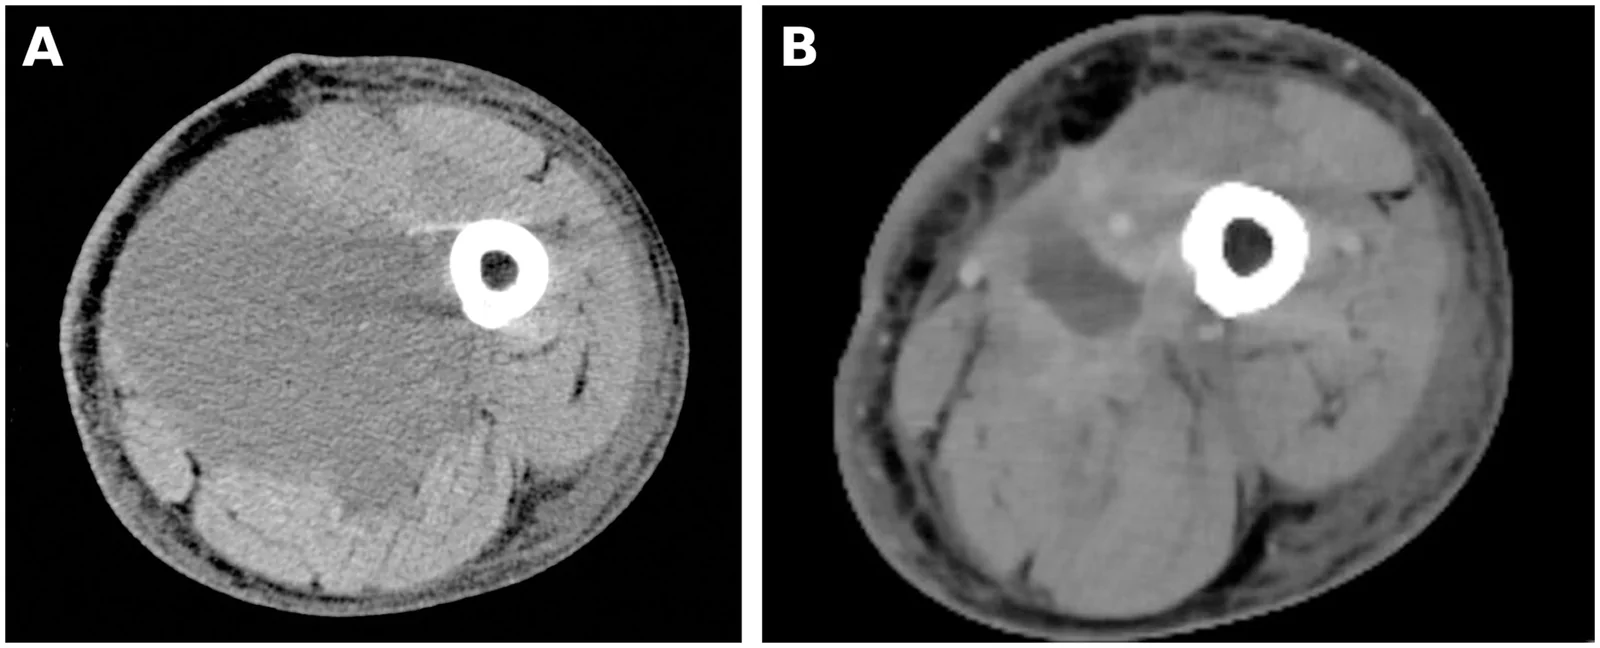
Axial CT comparison of the left thigh before and after D–LVA. (A) Preoperative CT showing diffuse lymphatic fluid accumulation with poor delineation of muscle planes. (B) Postoperative CT showing marked reduction in fluid and clear delineation of muscle compartments.
